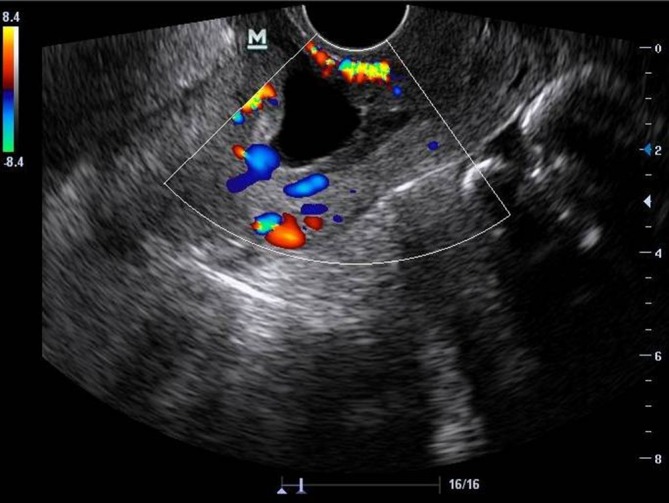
Colour Doppler imaging showing a rim of vascularity, representing a choriodecidual reaction compatible with ectopic pregnancy.
